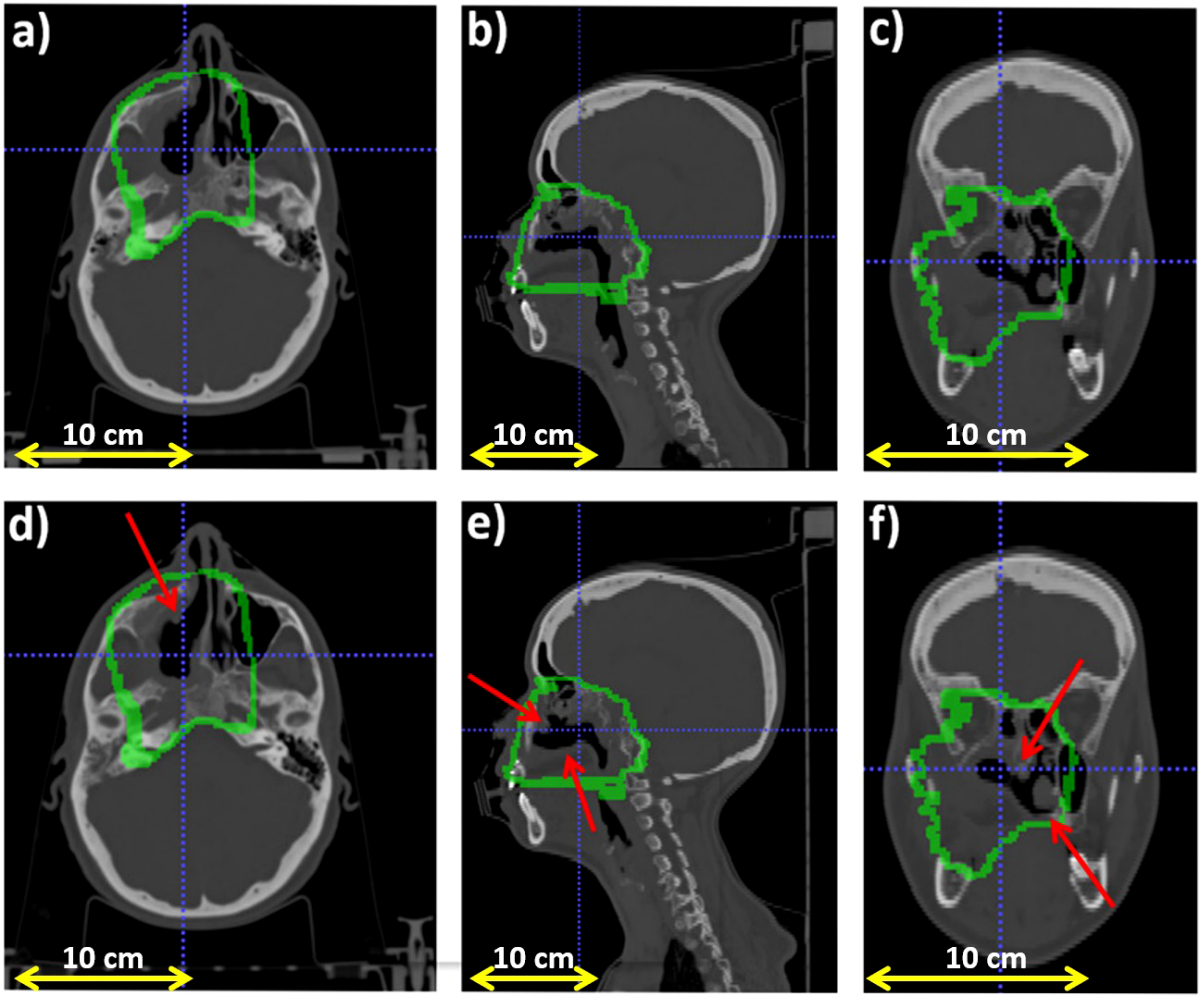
Planning CT (upper row) and control CT (lower row) for patient 007P. (A) Axial, (B) sagittal and (C) coronal views for the planning CT, and (D) Axial, (E) sagittal and (F) coronal views for the control CT. The morphological change is highlighted with a red arrows and the CTV (Clinical Target Volume) area is highlighted with a green line.
